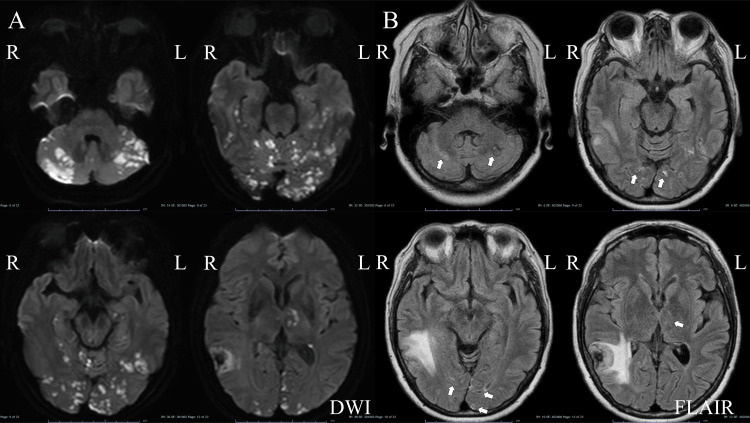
Magnetic resonance images obtained after middle meningeal artery embolization. Diffused ischemic lesions in the posterior cerebral lobes, cerebellar hemispheres, and thalami (A). FLAIR images obtained one year after ischemic complications (white arrow) (B). DWI: diffusion-weighted image, FLAIR: fluid-attenuated inversion recovery image.
